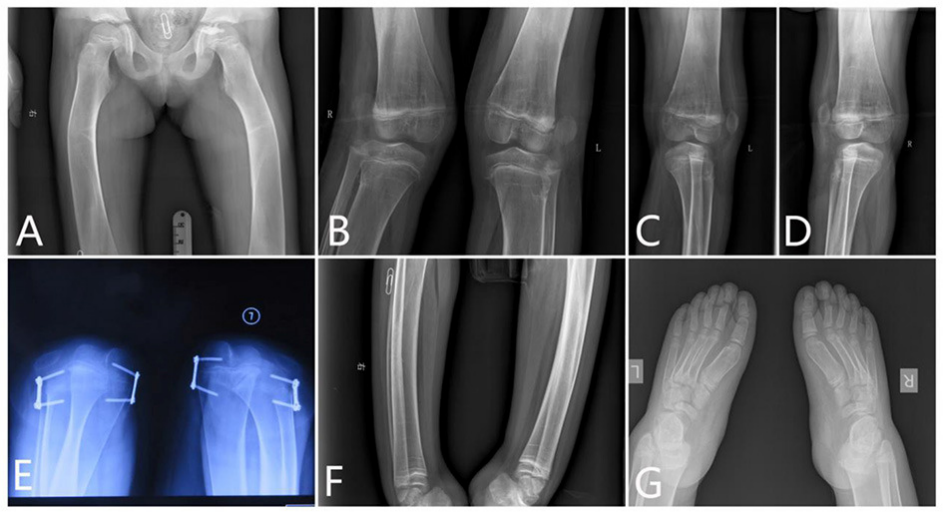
The x-ray radiography of the lower limbs. [(A) femurs, (B–D) knees, (E) shows lateral dislocation of the patella, (F) tibia, and (G) feet].
